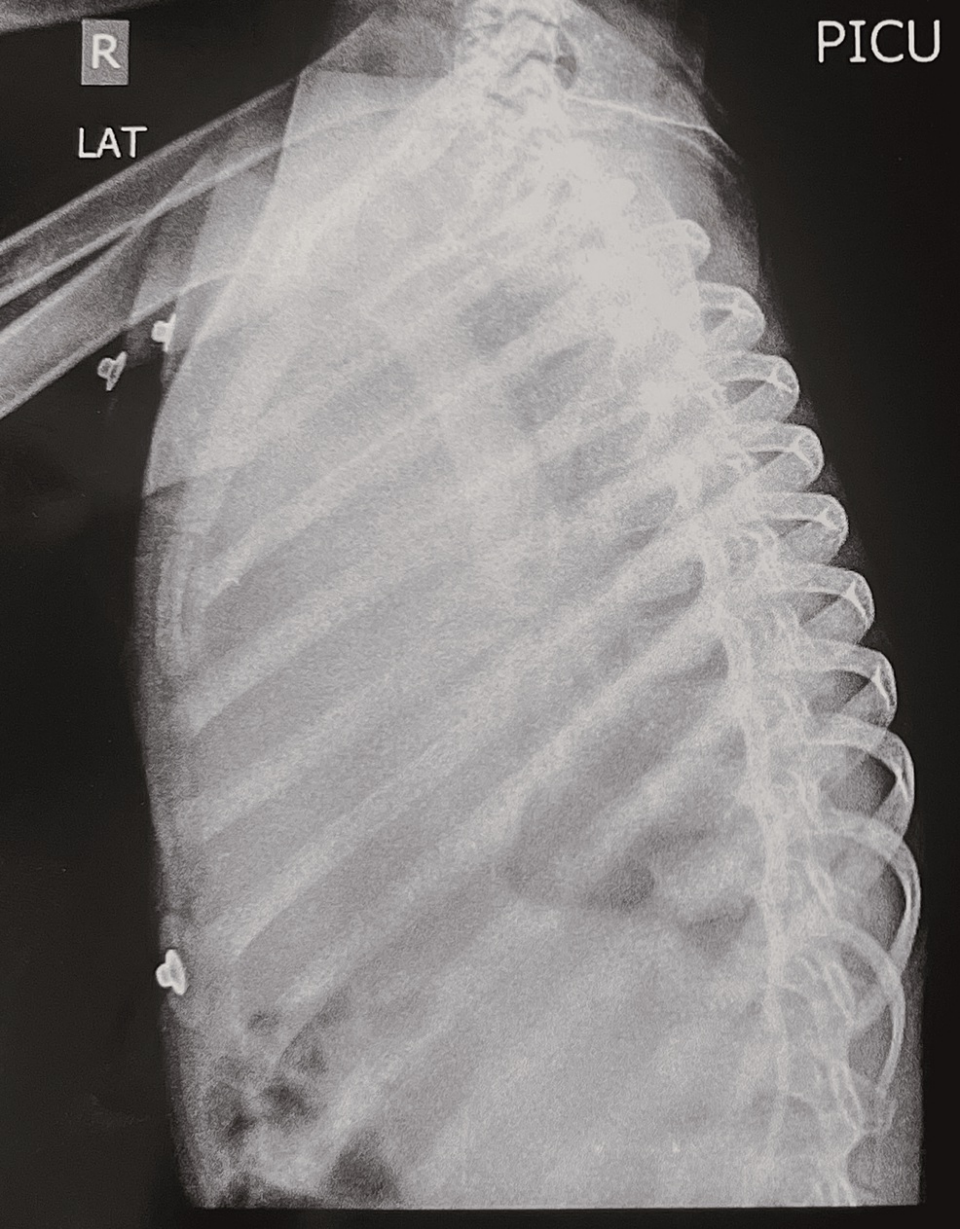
Plain chest X-ray in the right lateral decubitus view
